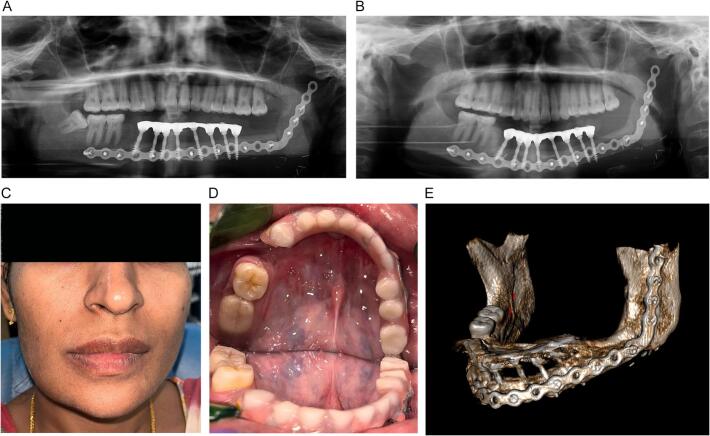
A. The post-operative panoramic radiograph at 6 months of follow-up. (Note: the healing of the flap.) B. The post-operative panoramic radiograph at the one-year follow-up visit (note: the healing of the flap). C. Patient's frontal view at 5-year follow-up. D. The intraoral view of the patient after 5 years of function (the image was taken using a mirror) E. A 3D cone beam CT view showing the complete union of the free fibula flap after 5 years of follow-up.
